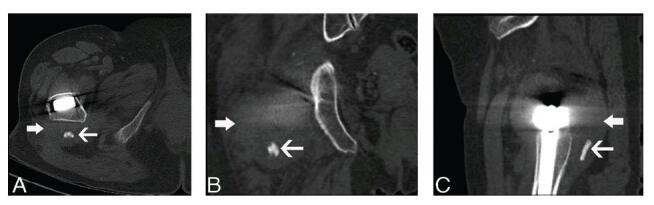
Figure 2. CT scan of the artificial hip before the first revision. A, Axial CT scan shows a mass-like lesion behind the prosthetic stem with a small amount of calcified tissue. B, Coronal CT scan shows a mass-like lesion behind the prosthetic stem with a small amount of calcified tissue. C, Sagittal CT scan shows a mass-like lesion behind the prosthetic stem with a small amount of calcified tissue. White thick arrow points to mass like lesion, and white thin arrow points to calcified tissue. CT = computed tomography.
